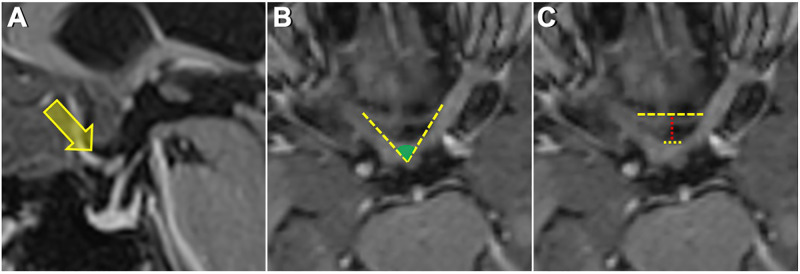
(A) Sagittal MRI image showing a prefixed chiasm—overlying tuberculum sella. (B) Measurement of the interneural angle on axial reformatted images along the intracranial optic nerves. (C) Upper dashed yellow line shows the distance between the bilateral optic nerves at the entrance to the optic canal. The lower dashed yellow line shows the distance between the optic nerves just before they form the optic chiasm. The dashed red line shows the distance from the anterior aspect of the optic chiasm to the limbus sphenoidale.
